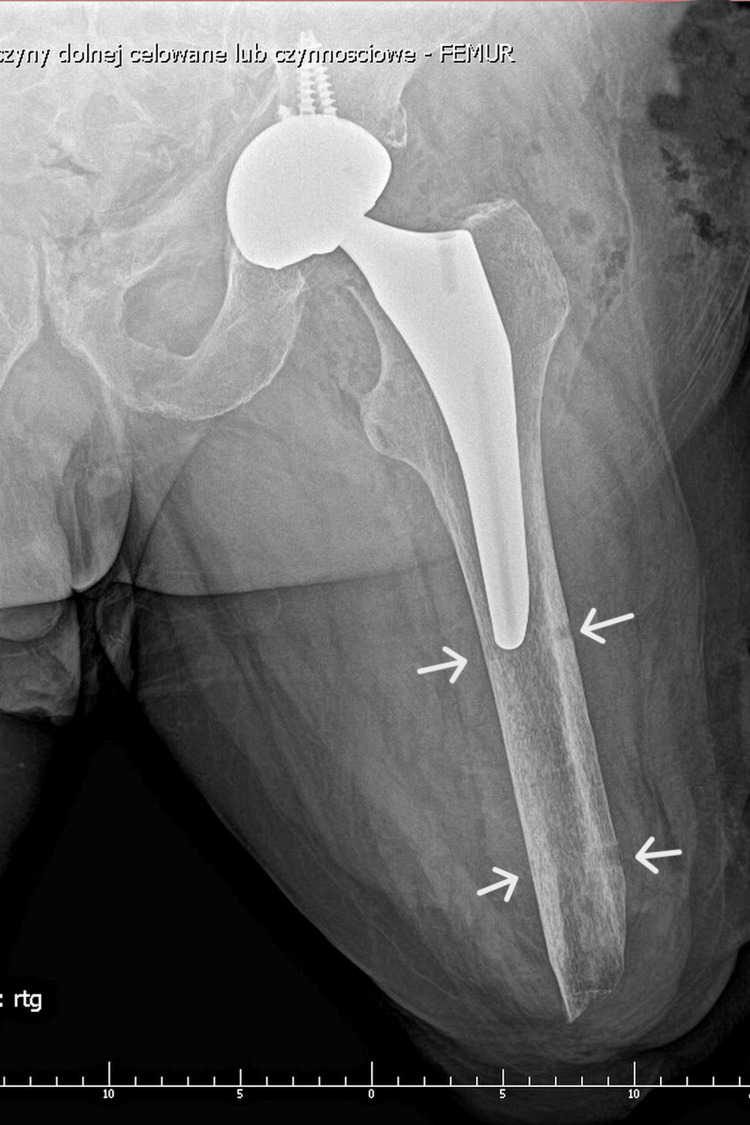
Immediate postoperative AP radiograph. Immediate postoperative AP radiograph showing stable acetabular and femoral components. Arrows indicate the sites where temporary rods were inserted into the residual femur to facilitate intraoperative manipulation.
